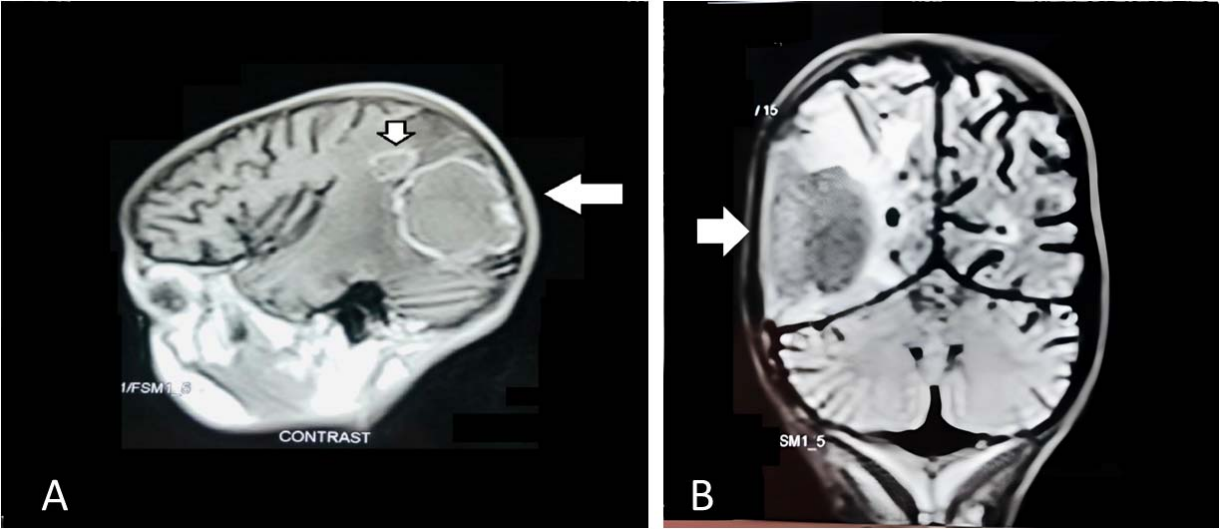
Magnetic resonance imaging of the brain in (A) sagittal and (B) coronal view showed a large ring-enhancing lesion (4.2 × 3.6 cm) in the right posterior temporal and parietal region with marked surrounding edema (horizontal arrow in A and B), as well as a small ring-enhancing lesion (1.3 × 1.4 cm) adjacent to the primary lesion (vertical arrow in A).
